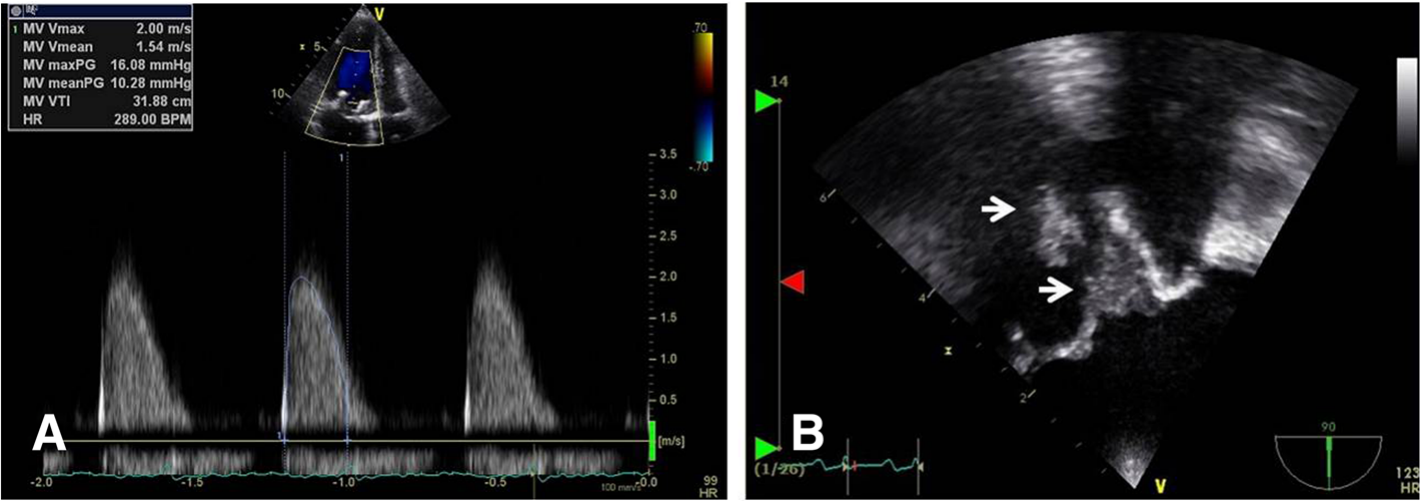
Transthoracic echocardiograms showing an elevated mean diastolic pressure gradient (a) of 10.3 mmHg and a hyperdynamic echogenic mass attached to the prosthetic MV (b) (*white arrows*)
